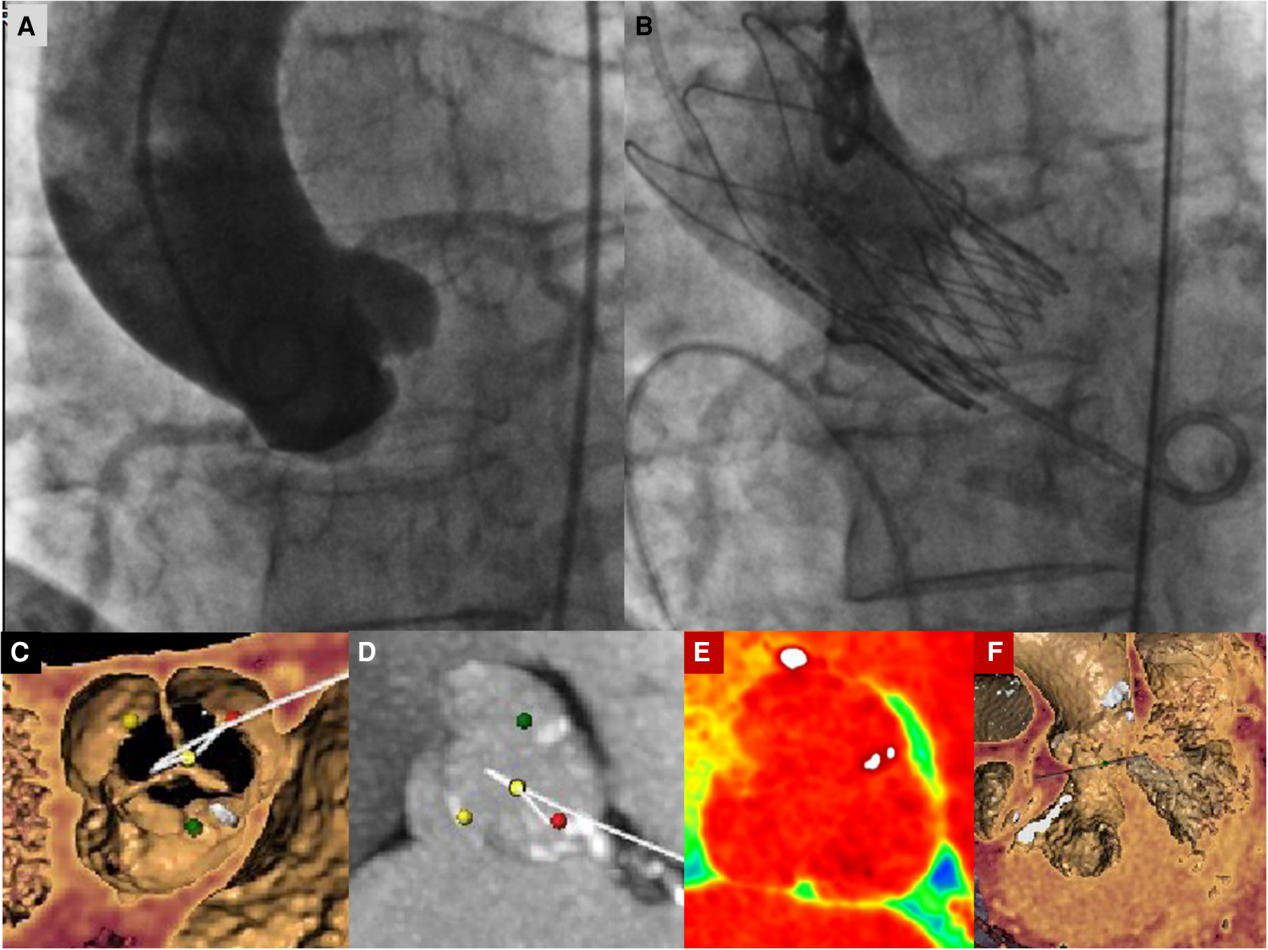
(A) Basal aortography in the “virtual basal plane” view (LAO 5°−10° cranial projection). (B) Final aortography documenting correct implantation of an Acurate Neo 2 small-size valve with patent coronary arteries and correct minimal LVOT protrusion of the THV to ensure anchoring without any trace of paravalvular leak. (C) Preprocedural images of CT angiography with tridimensional reconstruction of the aortic valve showing a tricuspid aortic valve. The yellow dot identifies the non-coronary cusp, the red dot identifies the left coronary cusp, and the green dot identifies the right coronary cusp. (D–F) Preprocedural images of CT angiography with tridimensional reconstruction of the aortic valve showing a tricuspid aortic valve without significant calcific degeneration and left and right ventricular chambers. In Panel (D), the yellow dot identifies the non-coronary cusp, the red dot identifies the left coronary cusp, and the green dot identifies the right coronary cusp.
